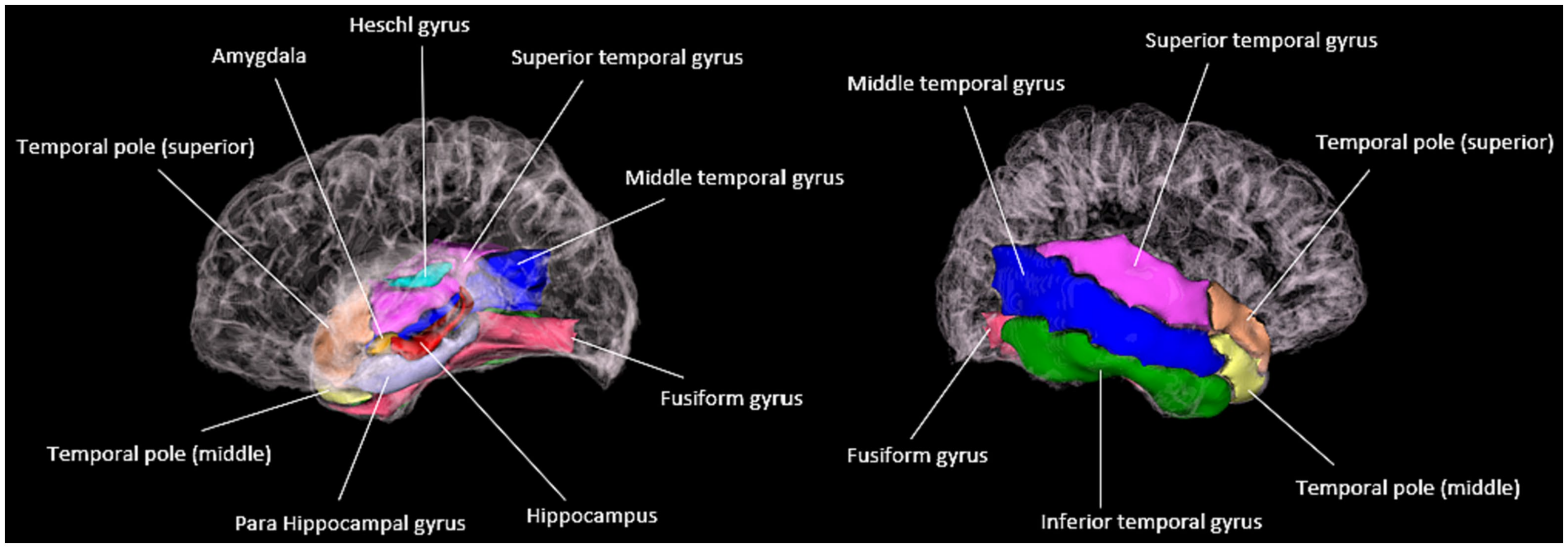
Visualization of TLE morphometric feature extraction from MR images.
